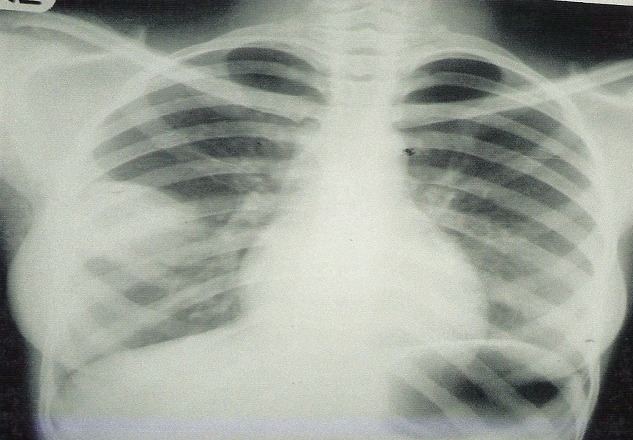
Frontal chest radiograph showing a fairly well marginated, rounded homogenous opacity in the lateral aspect of the right middle lung zone
